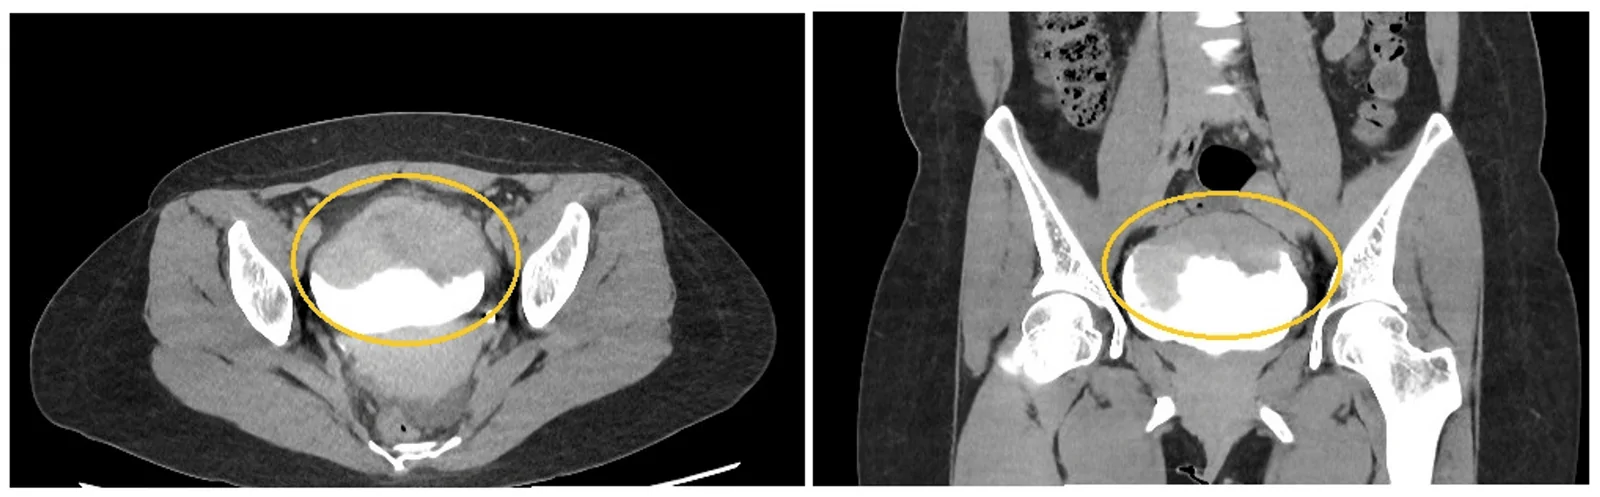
Initial contrast-enhanced CT demonstrating the invasive anterior bladder wall mass. The yellow circle indicates the bladder mass.
